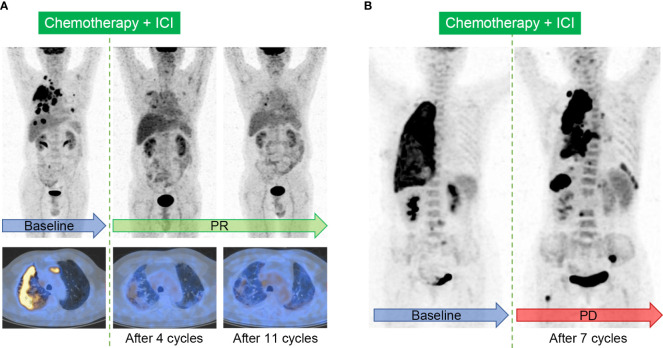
Typical cases evaluated by Response Evaluation Criteria in Solid Tumors (RECIST) 1.1 in patients with ICI treatment. (A), Immunotherapy response in a 64-year-old male patient with right lung adenocarcinoma. The baseline image shows intensive FDG uptake in the primary tumor, accompanied with multifocal intrapulmonary metastasis, lymphadenopathy, and the involvement of pleura. Follow-up images after 4 cycles and sequential 11 cycles of the combination of chemotherapy and ICI show partial response (PR). (B), Immunotherapy response in a 60-year-old female patient with right lung adenocarcinoma. Image after 7 cycles of the combination of chemotherapy and ICI shows the enlargement and increased metabolism in the primary tumor, and the onset of multiple new lesions in the lung, pleura, lymph nodes, liver, and bone, indicating progressive disease (PD).
